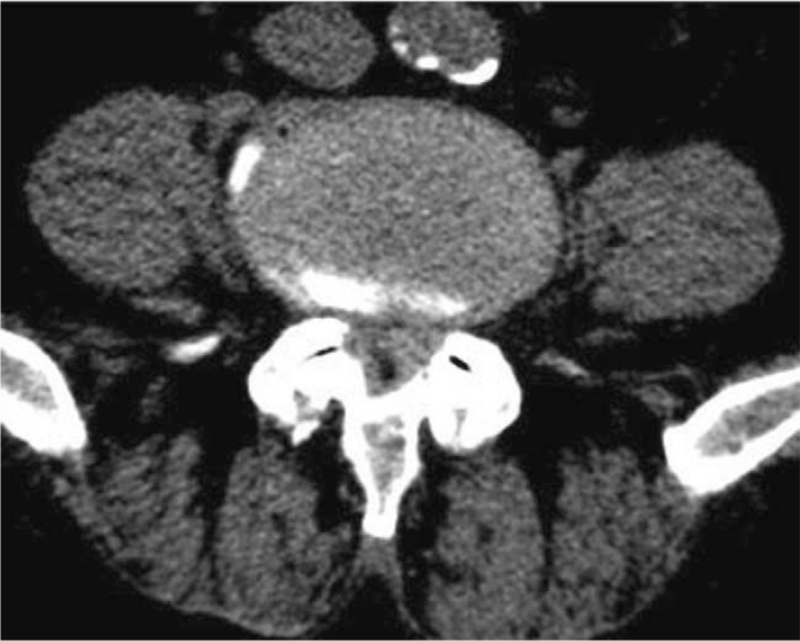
CT showing a posterior root and theca compression at L4-L5 intervertebral level.
